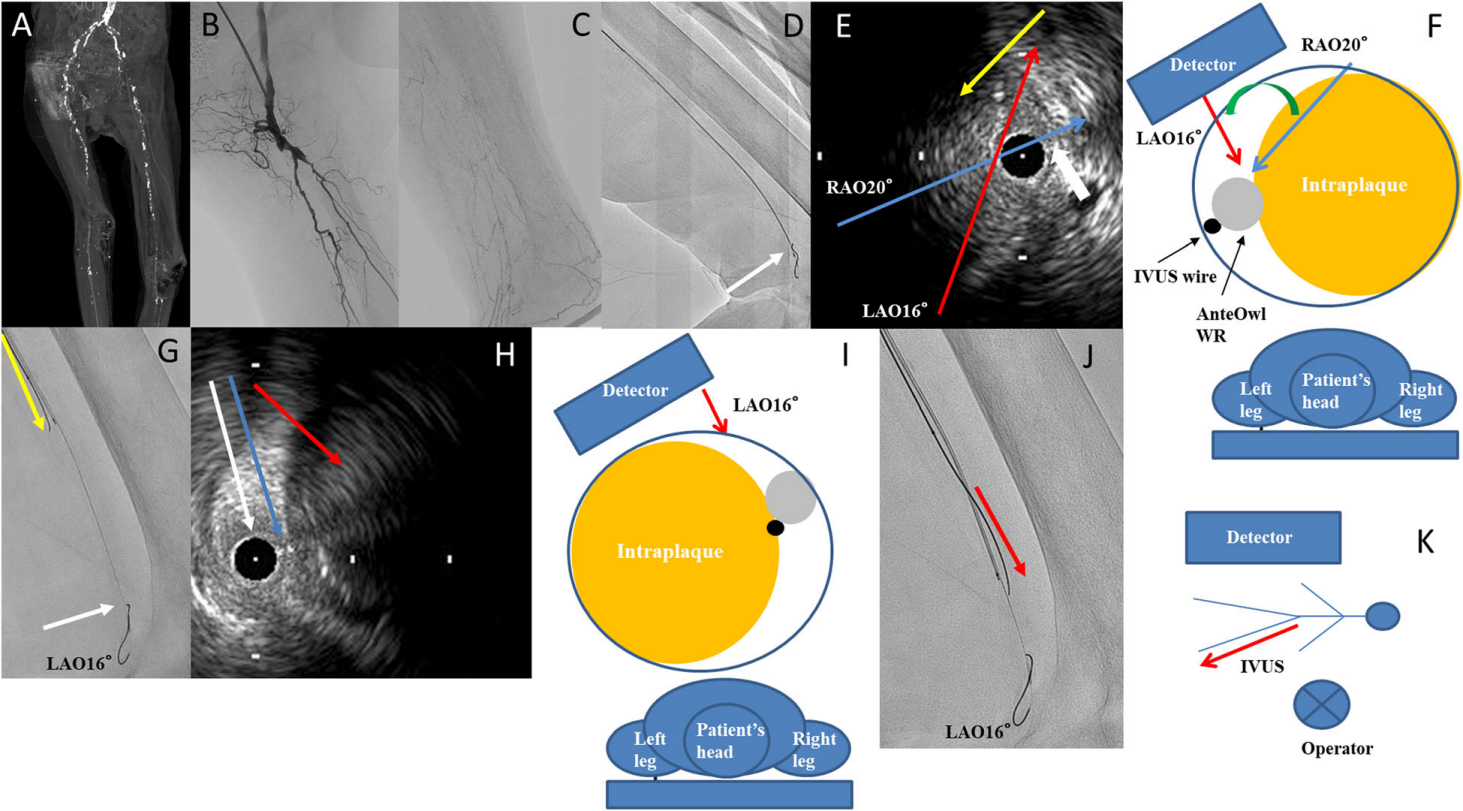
a Preprocedural enhanced computed tomography. b, c Control angiography showed total occlusion from the proximal aspect of the superficial femoral artery to the popliteal artery. The distal true lumen of the popliteal artery was minute (white arrow). d AnteOwl WR intravascular ultrasound (AnteOwl IVUS) could be advanced to the popliteal artery (white arrow). e IVUS findings of a superficial femoral artery distal lesion. The IVUS catheter was in the subintimal space. The white arrow shows the IVUS wire. The IVUS wire and transducer coincided with right anterior oblique 20° (blue arrow), and left anterior oblique 16° was maximally separated of IVUS and target plaque (red arrow). The yellow arrow shows that the direction of the second guidewire should be advanced. f Cross-sectional image from the proximal position (operator position). The transducer was on the surface side and the target plaque was on the right side of the IVUS catheter. g We converted the intravascular ultrasound (IVUS) findings into an angiographic image. The IVUS wire was on the right side (left anterior oblique side), the transducer was in the center, and the second guidewire was on the left side (right anterior oblique side) on angiography; these findings are almost the same as the IVUS findings in e White arrow shows the tip of AnteOwl WR IVUS. h, i IVUS findings of the popliteal artery lesion. The blue arrow shows the IVUS wire. The yellow arrow shows the IVUS transducer. The red arrow shows the direction of the target plaque at which we aimed. The target plaque was on the left side of the IVUS catheter. j The IVUS wire was in the center, the transducer was on the left side, the second guidewire was on the right side (left anterior oblique side) on angiography; these findings are almost the same as the IVUS findings. k Positional relationship between patient and operator and direction of IVUS
